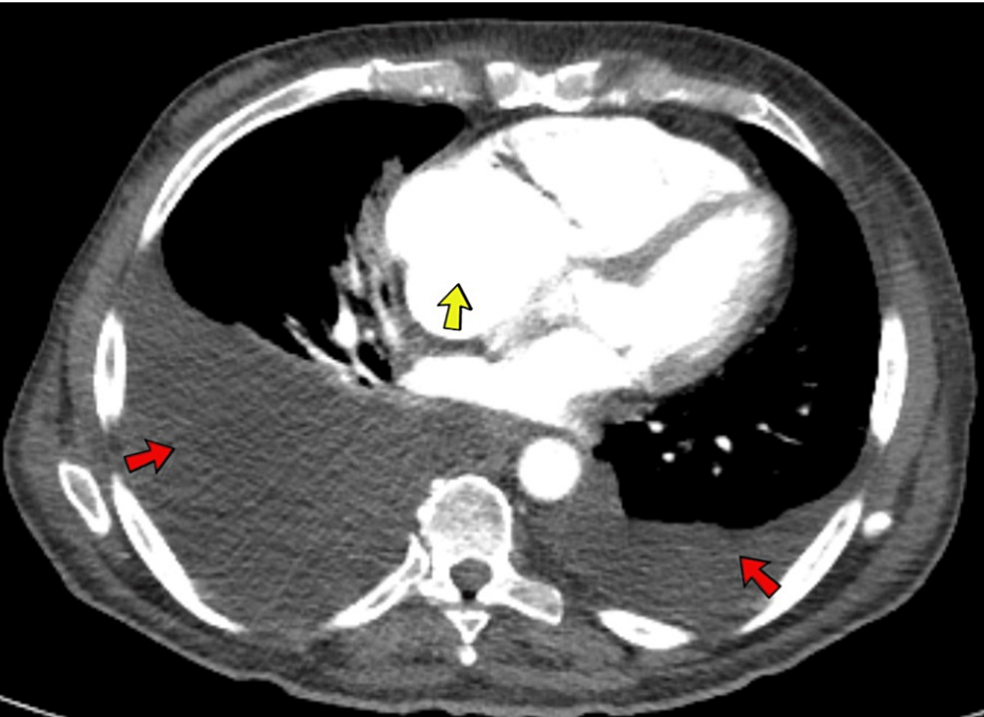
A CT scan of the chest, abdomen, and pelvis showed large right-sided pleural effusions and small to moderate-sized left-sided pleural effusions (red arrows). Increased right atrial size (yellow arrow) with a borderline enlarged heart.
